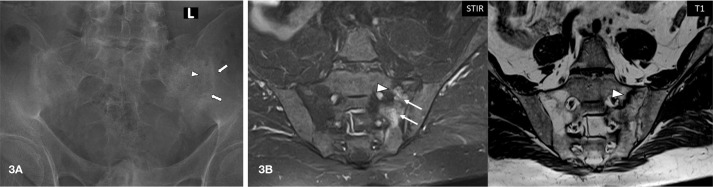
(A) Radiograph of the pelvis from a patient with low back pain and structural SIJ findings indicating post-inflammatory changes (arrows) and suspicion of fracture (arrowhead). (B) MRI of the same patient with unilateral sacral insufficiency fracture on the left SIJ. The oblique coronal T1-weighted image shows a typical hypointense fracture-line (arrowhead), with surrounding bone marrow oedema, shown as hyperintense signal on STIR (white arrows)
